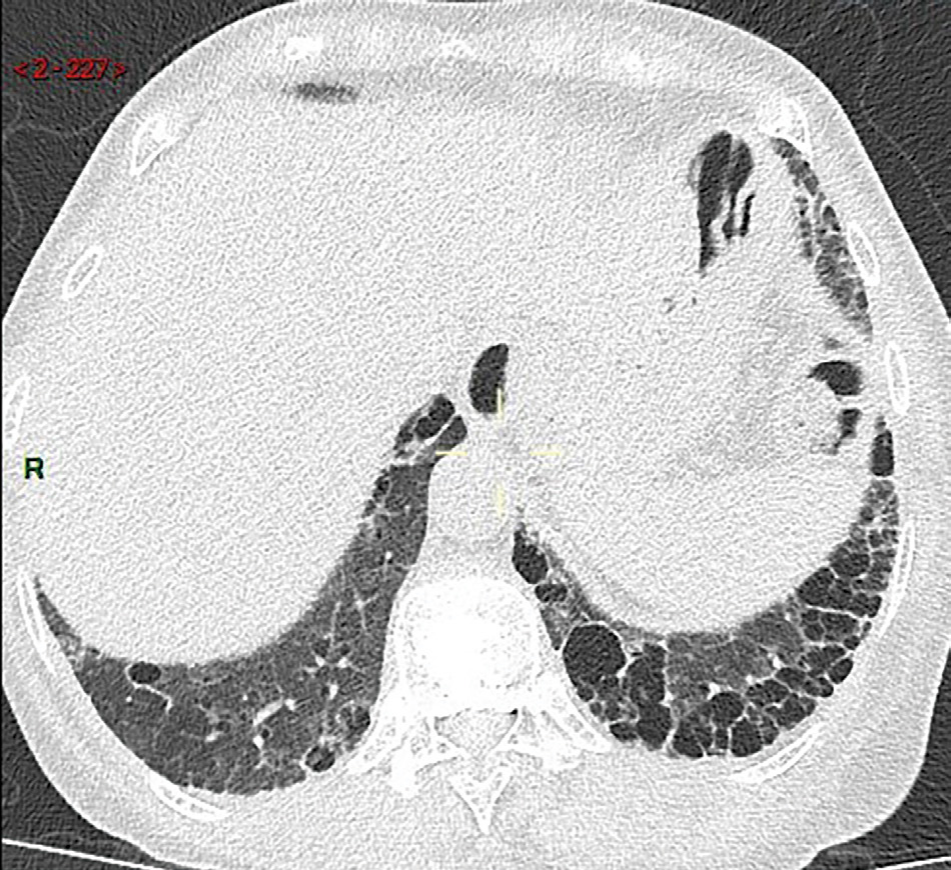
Axial follow-up CT image of lungs showing exuberant and macrocystic honeycomb at the periphery of the pulmonary bases, increasing compared to previous exam.
